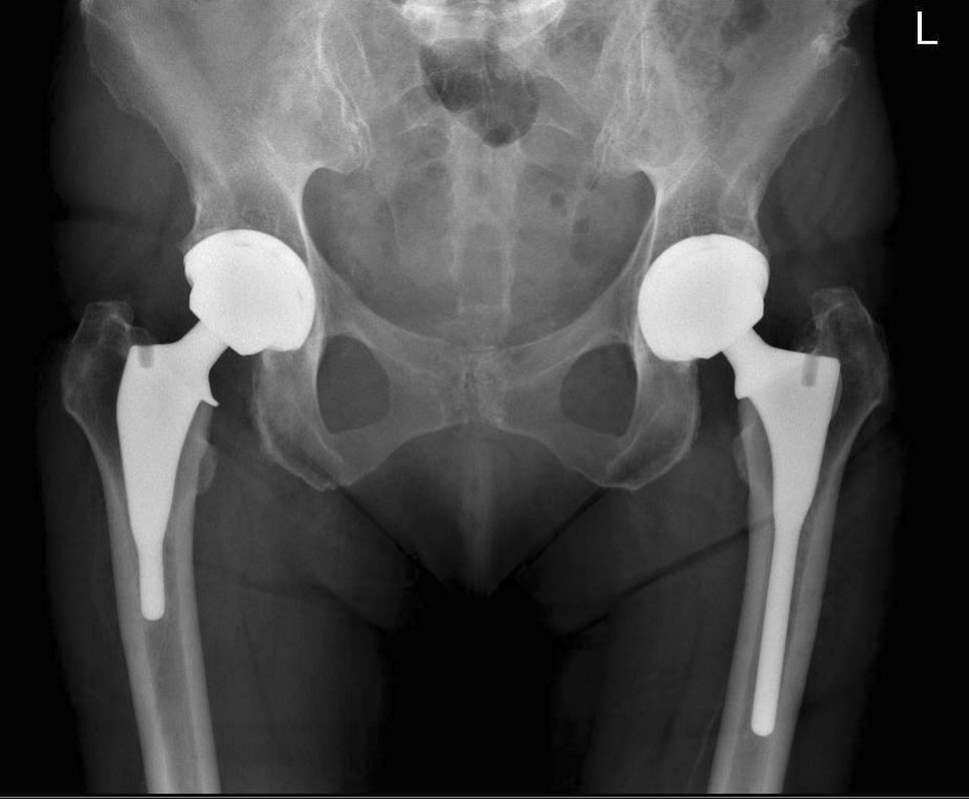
A postoperative weight bearing plain radiograph demonstrating the difference of femoral stem lengths
